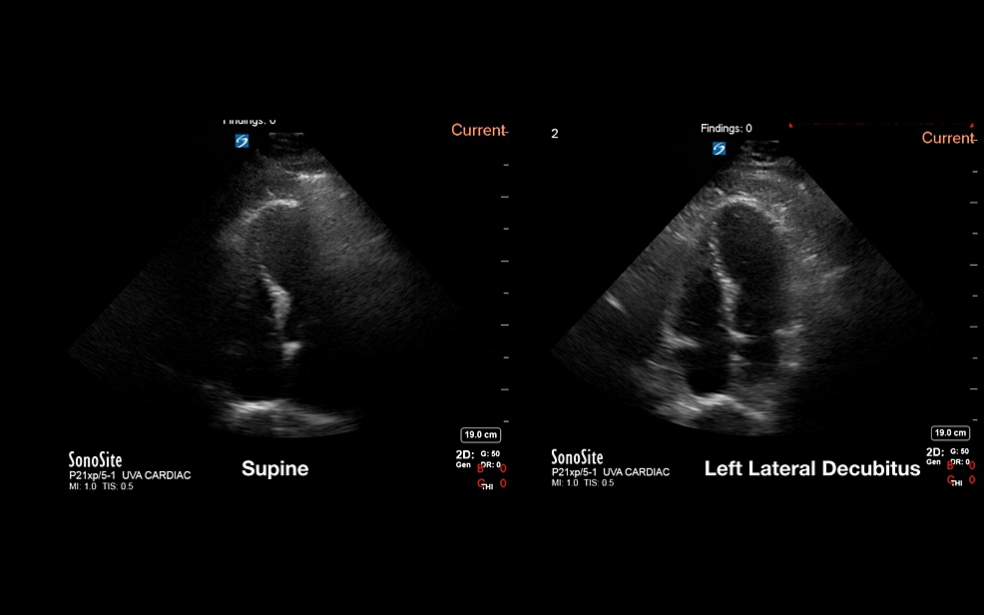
Example of image quality difference in the apical four window between the supine and left lateral decubitus positions within the same patient
